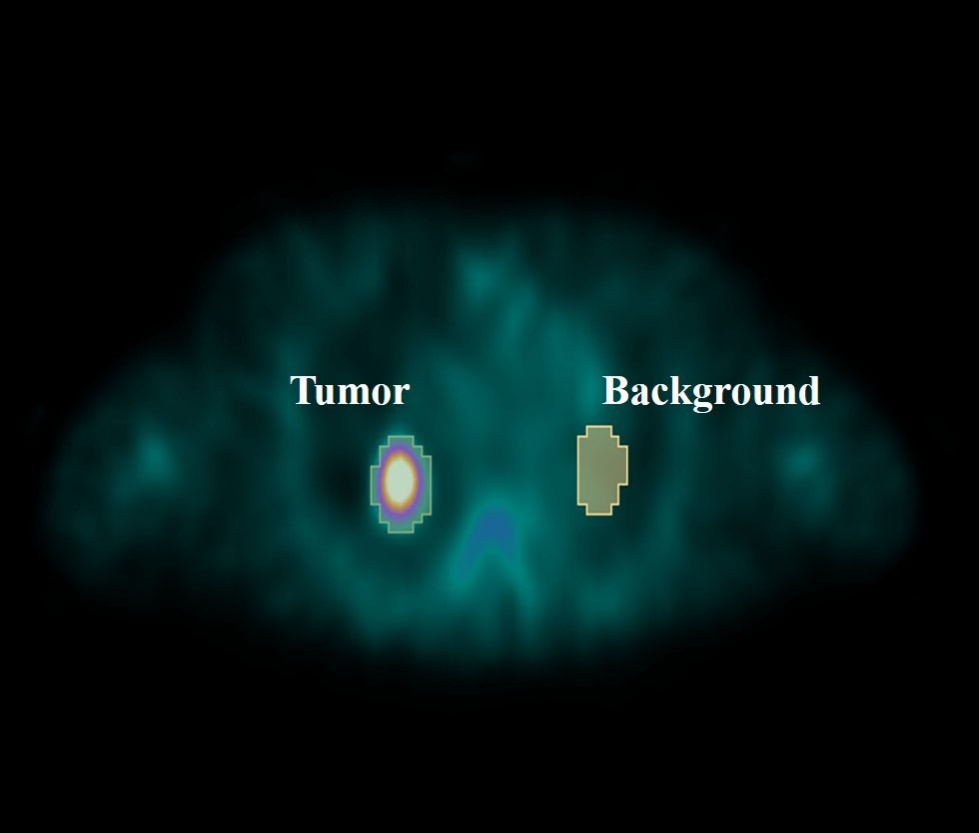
^99m^Tc-3PRGD_2_ chest SPECT images of the same patient based on NAC, CT-AC, and DL-AC. The top row are SPECT axial images (NAC, CT-AC, and DL-AC), and the bottom row are SPECT coronal images (NAC, CT-AC, and DL-AC). It can be seen that DL-AC significantly improves the contrast of the chest image, and the corrected image is close to the CT-AC image. On the axial image, the maximum diameter of the patient’s lung lesion was 49.98 mm, and the maximum T/B of the lesion on NAC, CT-AC, and DL-AC were 2.91, 3.80, and 3.78, respectively.
